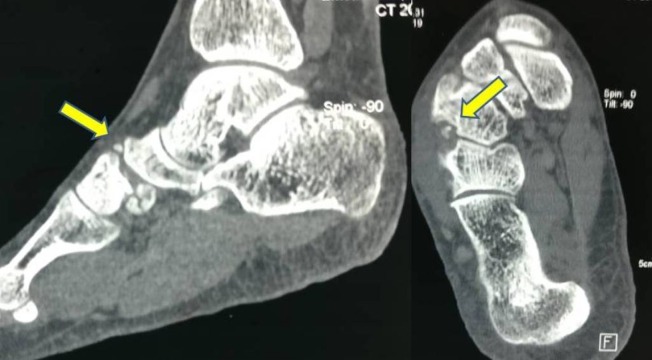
Computed tomography (CT) Shows arthritic first, second, and fourth TMT joints with osteophytes.
